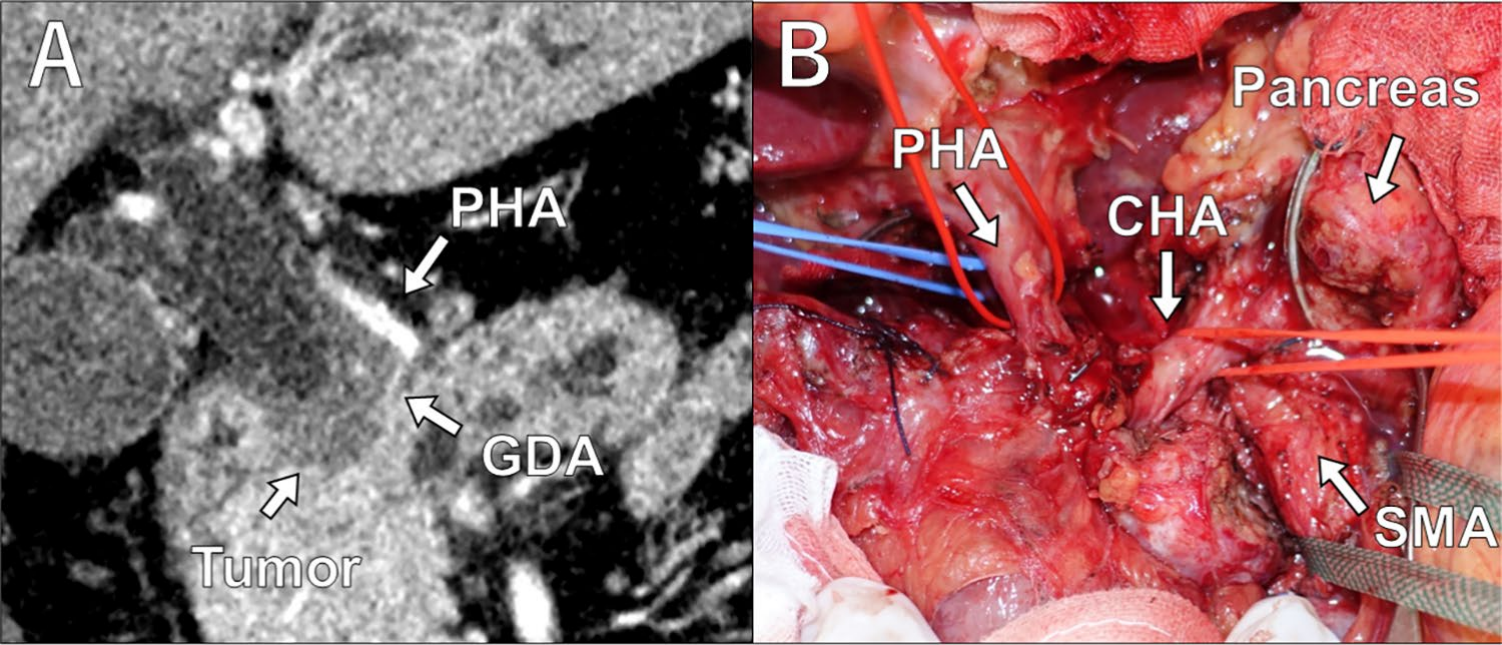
A Preoperative computed tomography images demonstrative of pancreatic head cancer in contact with the common hepatic artery (CHA), gastroduodenal artery (GDA), and proper hepatic artery (PHA). B Intraoperative findings indicate arterial involvement to the CHA, GDA, and PHA
